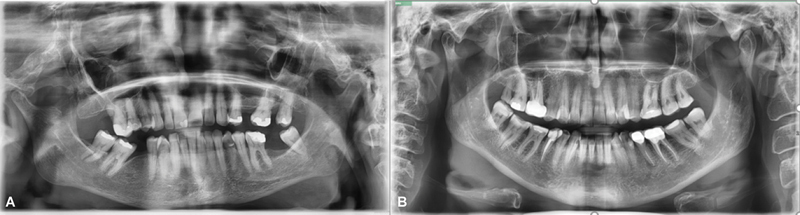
Panoramic radiographs of two 61-year-old female patients: (
